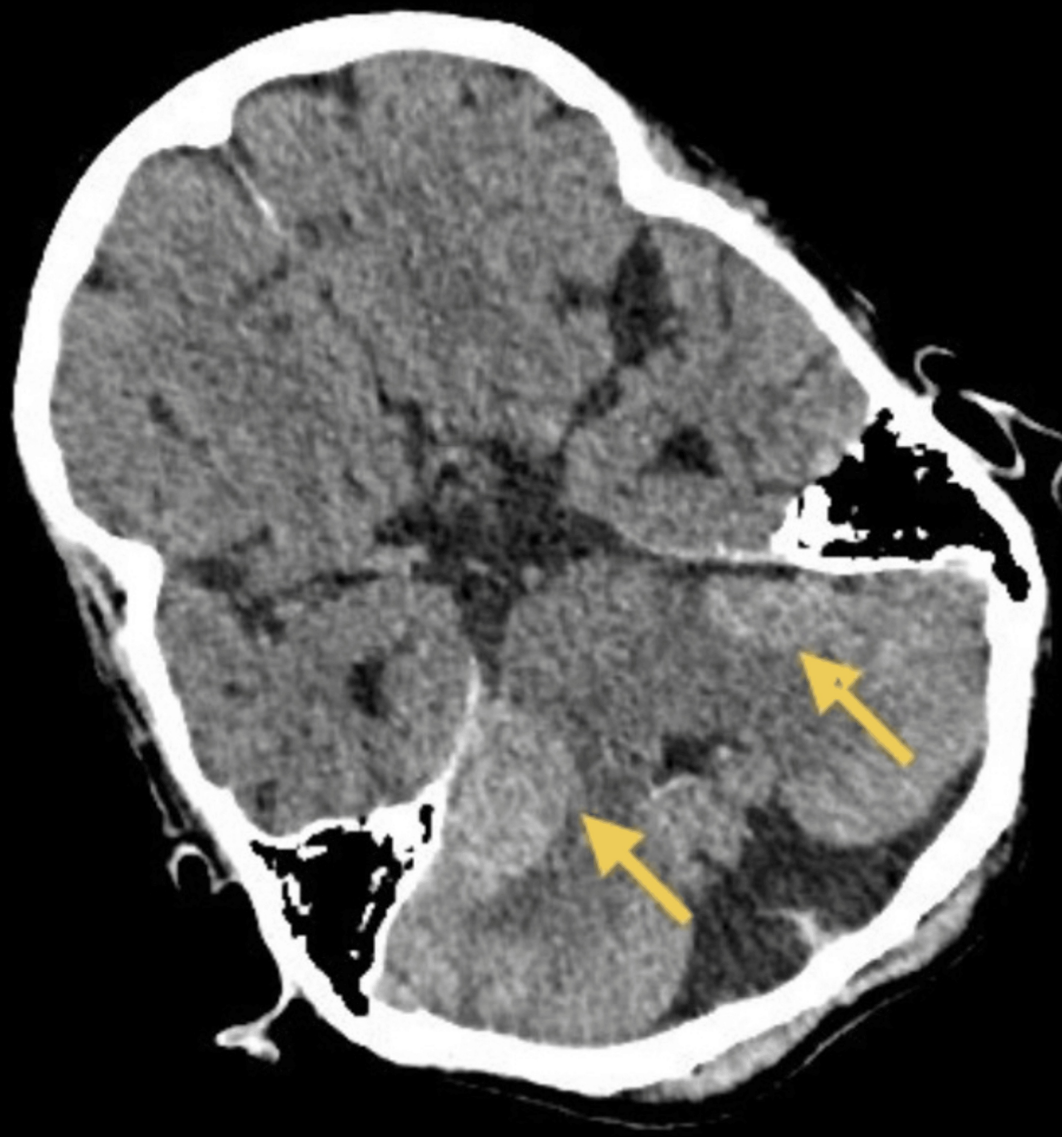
Contrast axial CT scan showing drastic increase in the size of bilateral cerebellopontine angle abnormalities. This contrast-enhanced CT image shows bilateral cerebellopontine angle lesion enlargement with cerebral oedema and ventricular dilatation. These findings contributed to the diagnostic uncertainty in the absence of an MRI.
